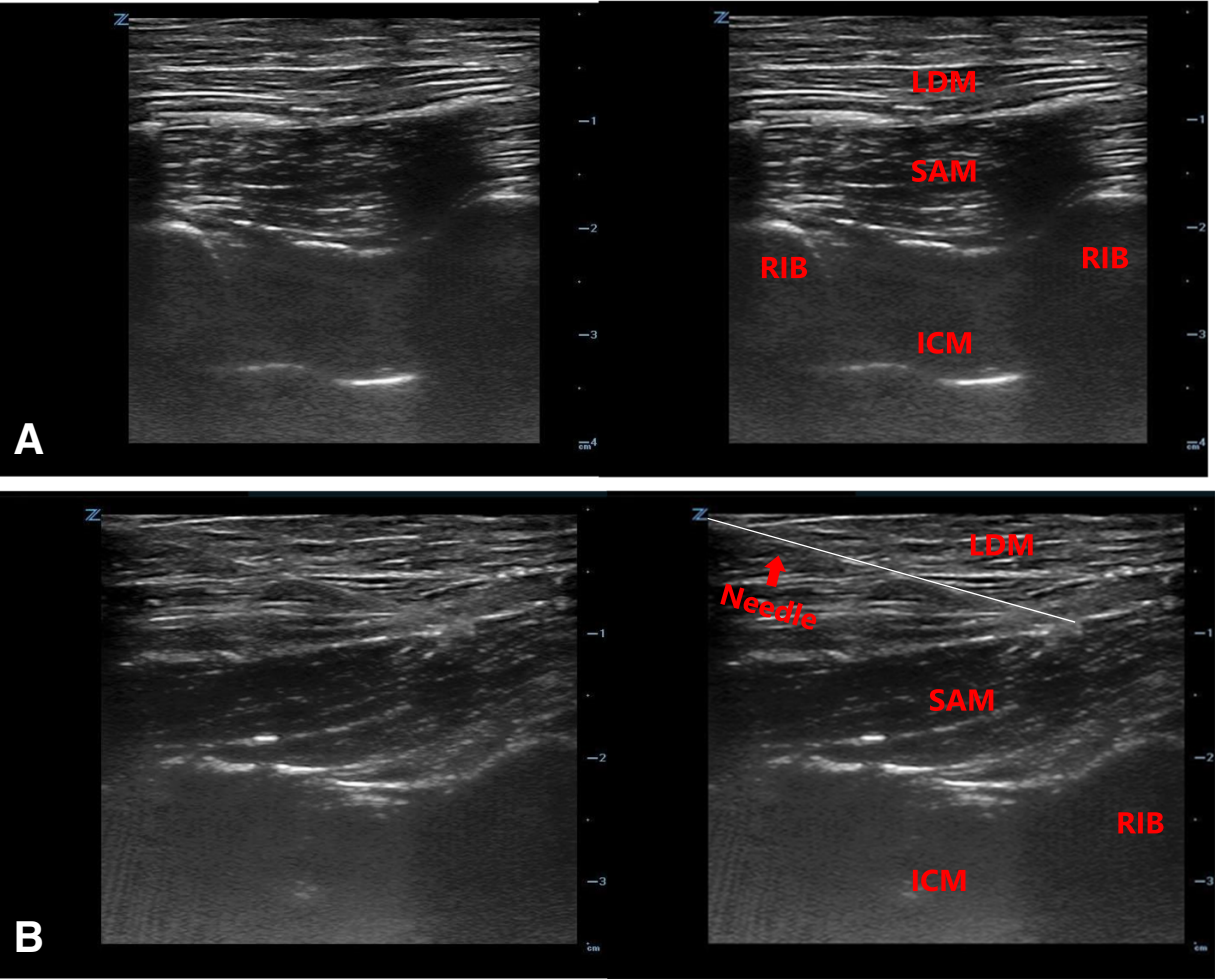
Ultrasound image of the serratus anterior plane model (a). Ultrasound image of needle placement (b)
